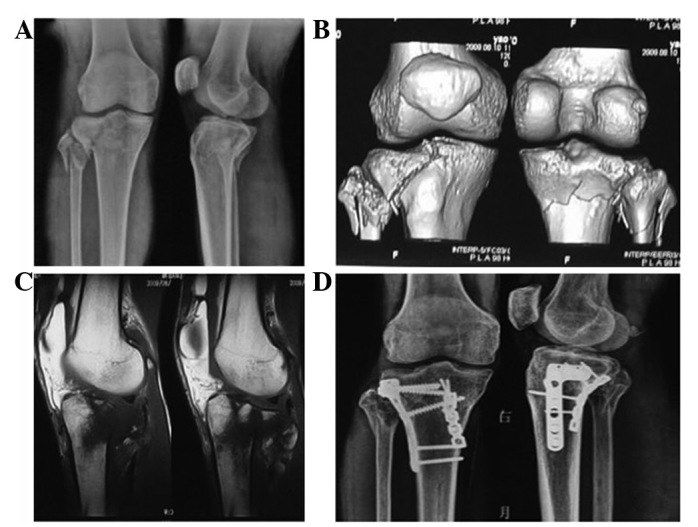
Male, 39 years old, with a tibial plateau fracture of the right tibial platform and a comminuted fracture of the small head of the fibula. (A) Preoperative X-ray. (B) Preoperative computed tomography (CT) and 3D reconstruction showing the comminuted fracture of the right tibial plateau, the collapse site in the lateral and posterior plateau and the comminuted fracture of the small head of the fibula. (C) Preoperative magnetic resonance imaging (MRI) showing the comminuted fracture of the right tibial plateau. The interior and posterior plateau collapses were serious. (D) One year following surgery, the X-ray showed that the right tibial plateau fracture fixation was good and that the joint surface was flat.
